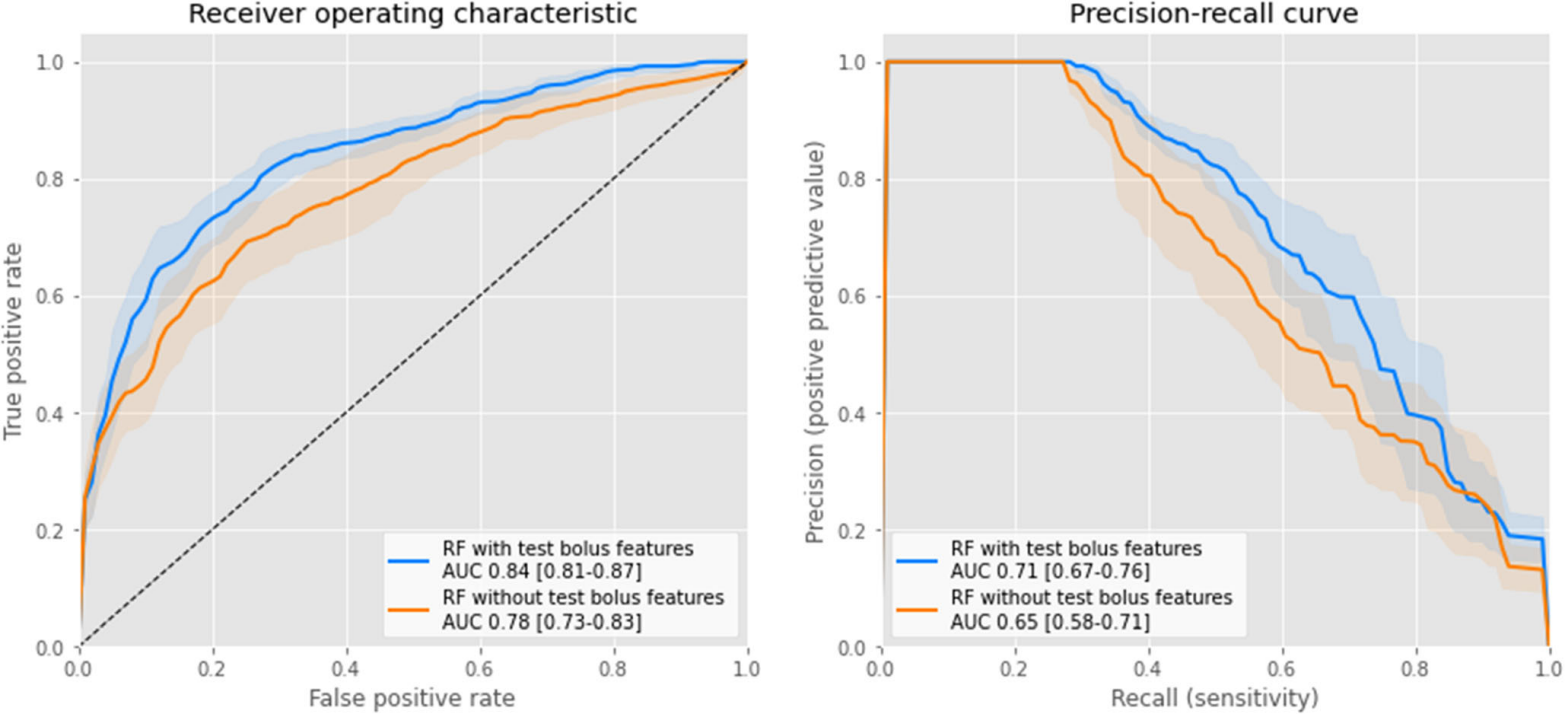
Average receiver operating characteristic (left) and precision-recall curves (right) with 95% confidence interval for models trained with patient features, CT settings, and test bolus features and with only patient features and CT settings. RF = *random forest*, *AUC = area under the curve*
